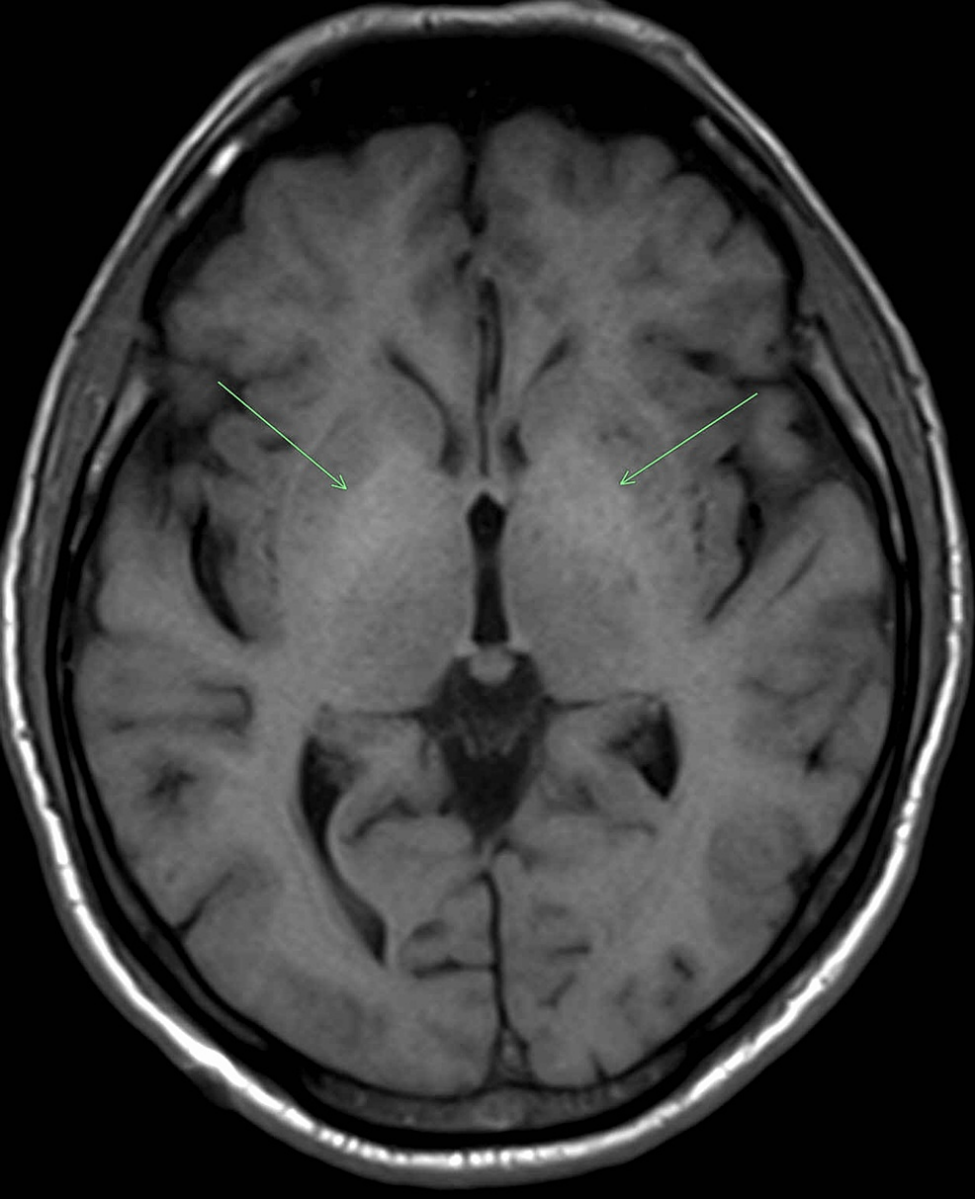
MRI brain in the axial view demonstrating subtle pre-contrast T1 hyperintensity in the bilateral basal ganglia (green arrows).
